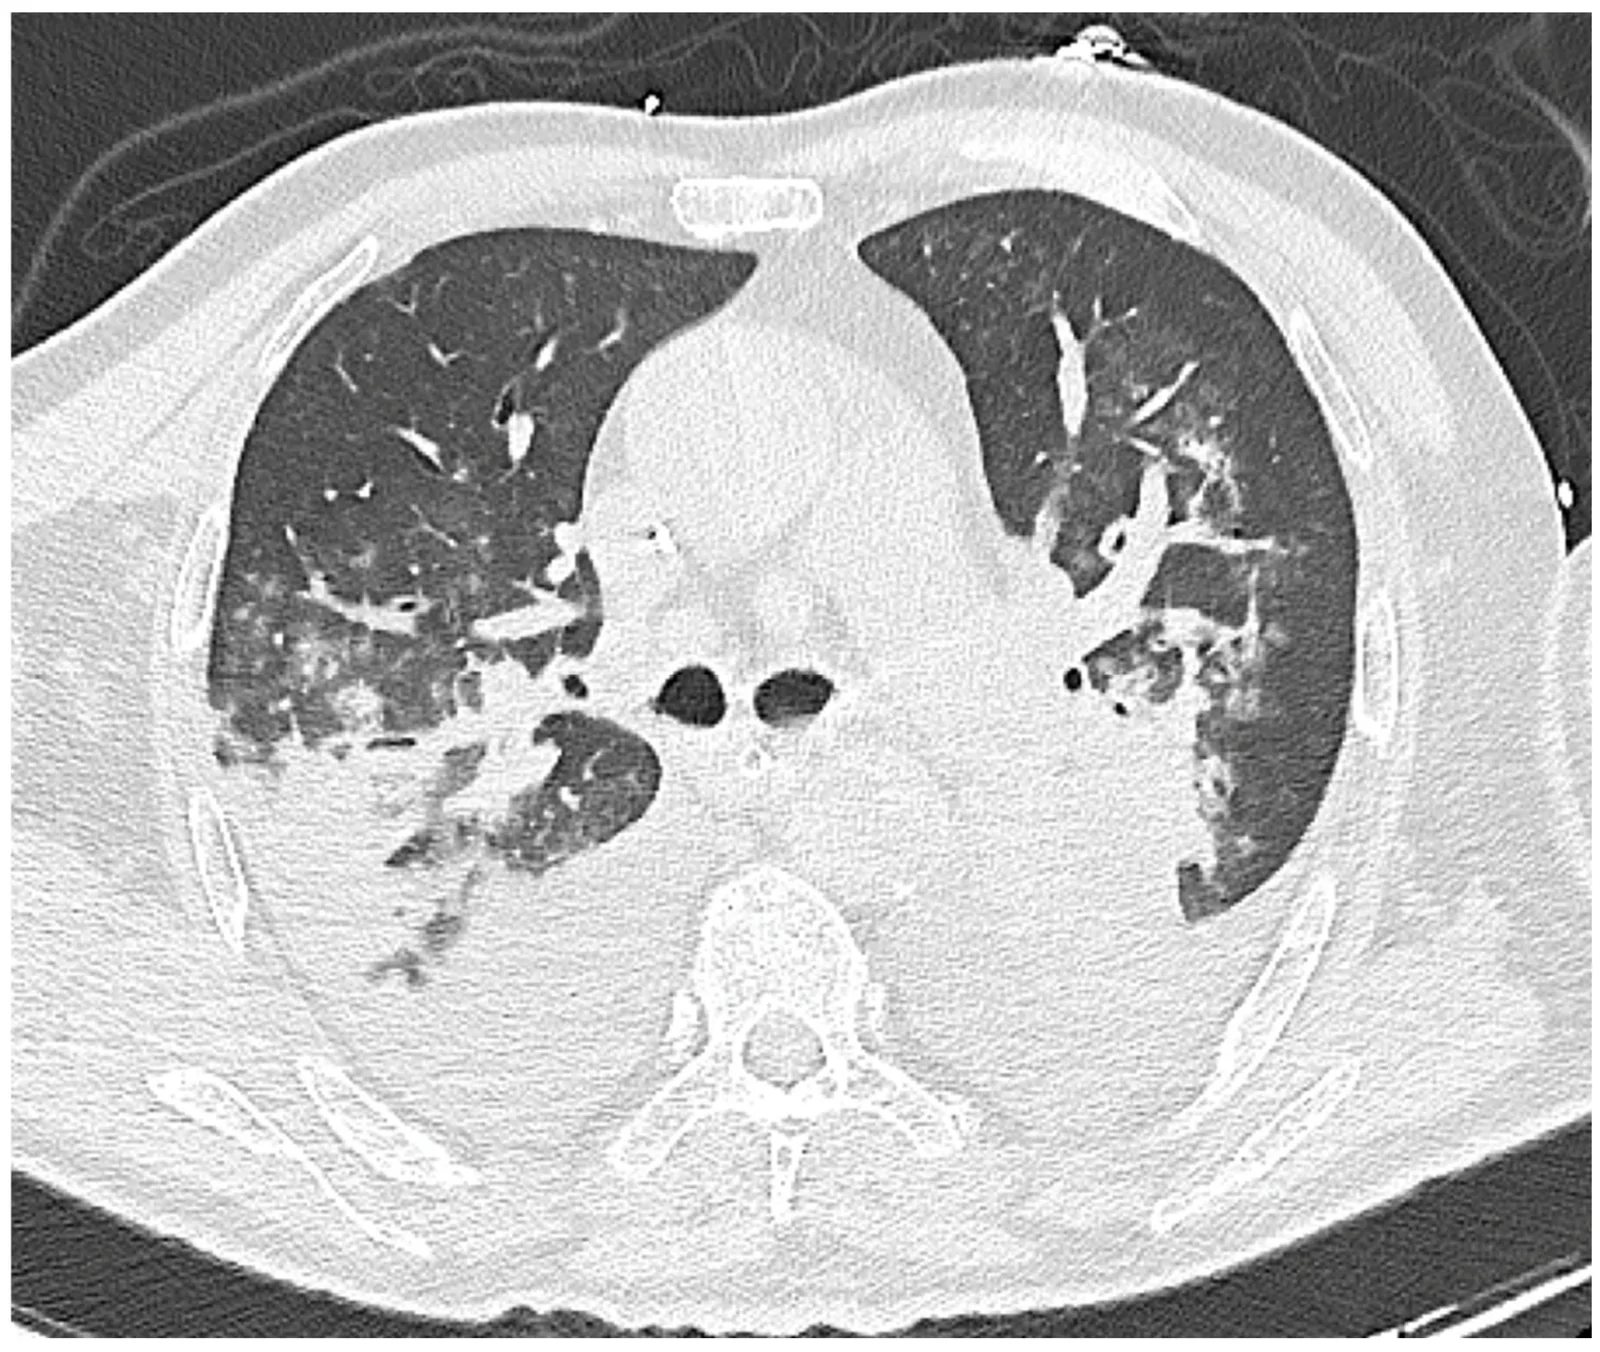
HRCT, axial plane. CT scans show an acute and advanced case of *Nocardia* lung infection, in presence of multilobar consolidations, GGO, and bilateral pleural effusion.
